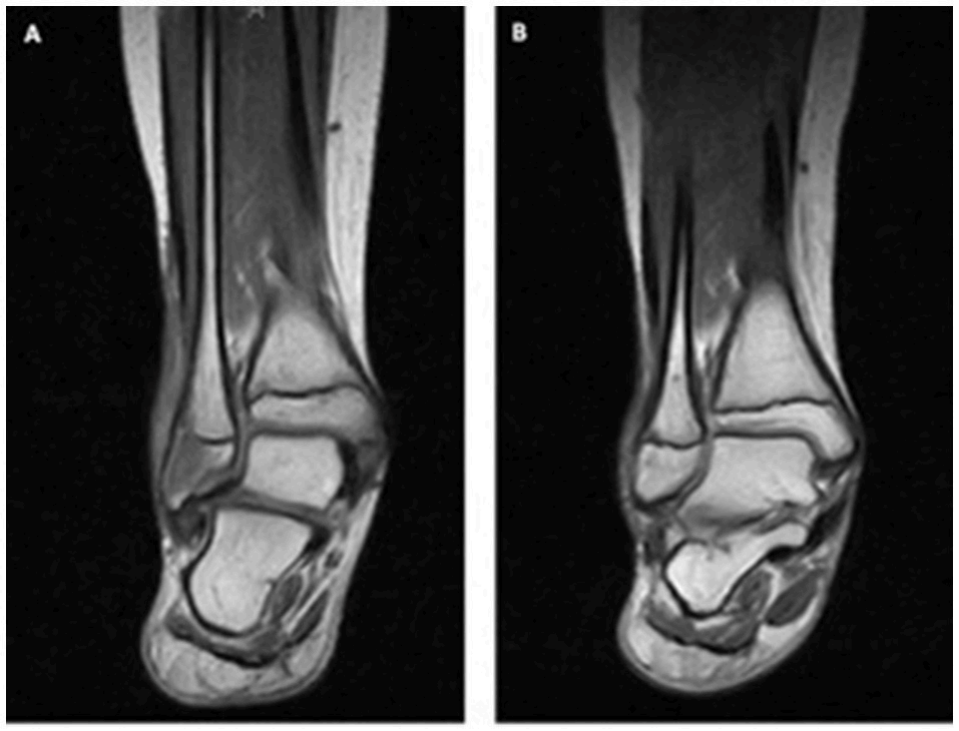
Granulomas in the bone of patient 7. MRI (A) at the beginning and (B) after 1 year of TNF inhibitor treatment.
